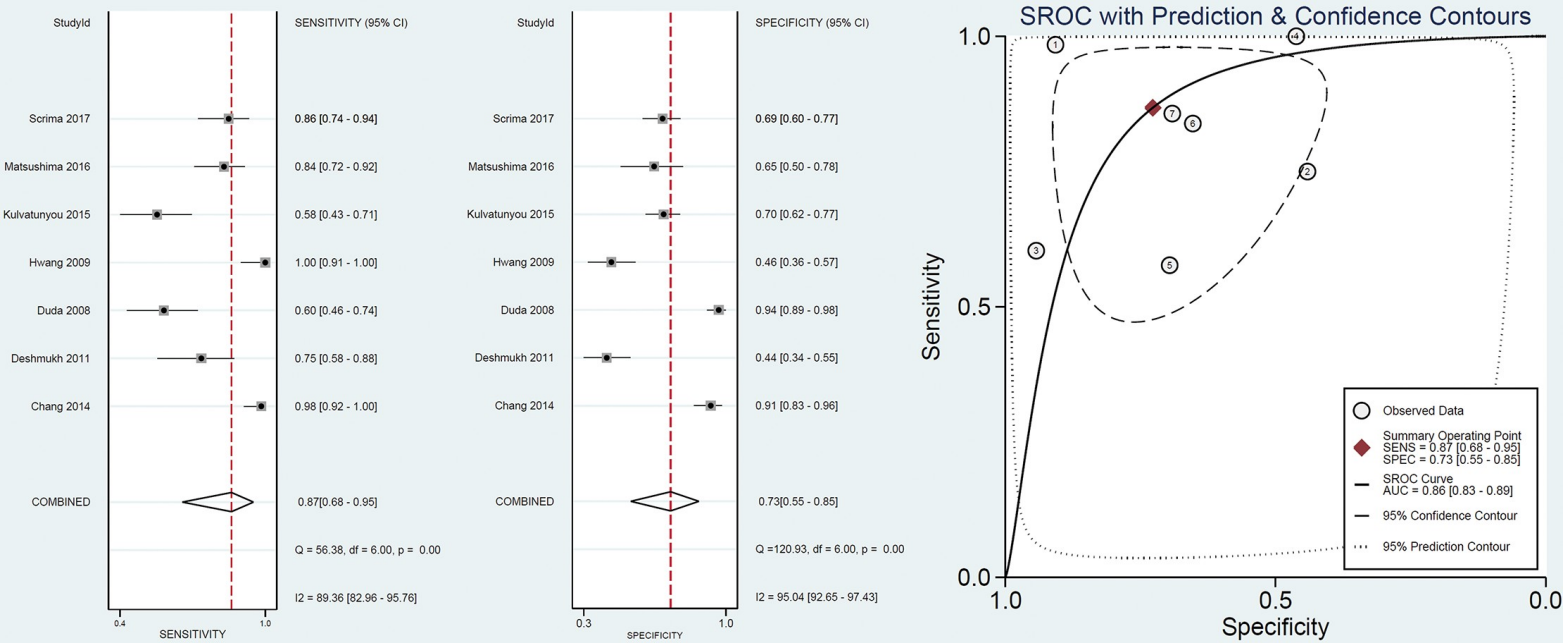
Value of CT in predicting surgical intervention.
